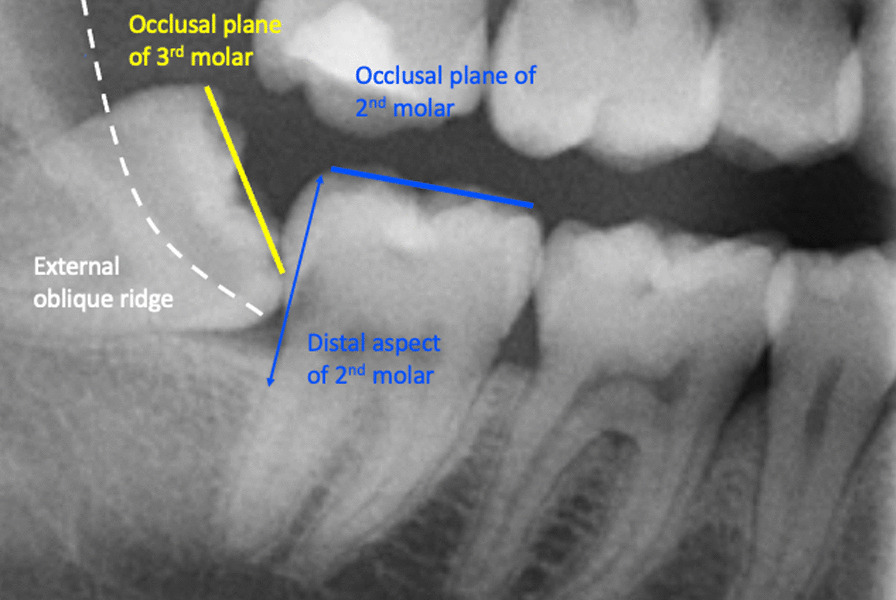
Indication of various anatomical points required for the assessment of third molar partial emergence/eruption on a panorama radiograph
